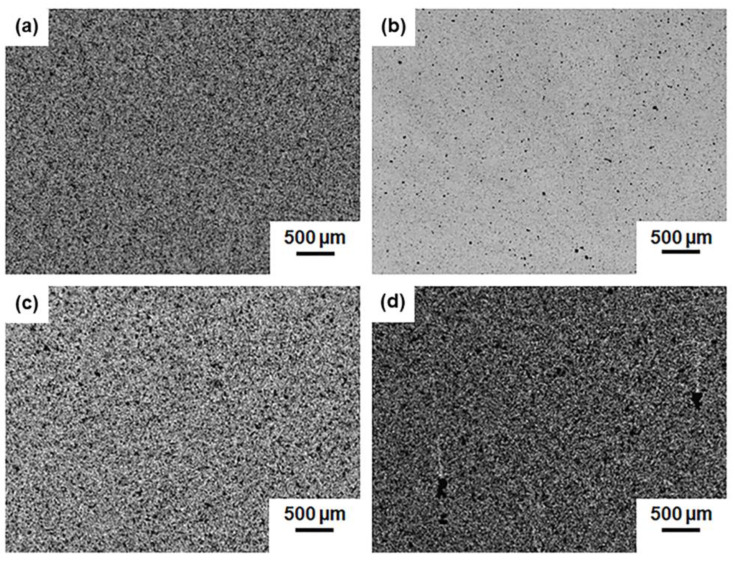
Optical images of (a) epoxy/GNP AO-4 (I_d_ = 0.532 ± 0.008), (b) epoxy/xGNP-C750 (I_d_ = 0.509 ± 0.015), (c) epoxy/xGNP-H5 (I_d_ = 0.478 ± 0.018) and (d) epoxy/xGNP-M5 (I_d_ = 0.437 ± 0.013) mixtures after sonication in ultrasonic bath at 80 °C for 90 min.
